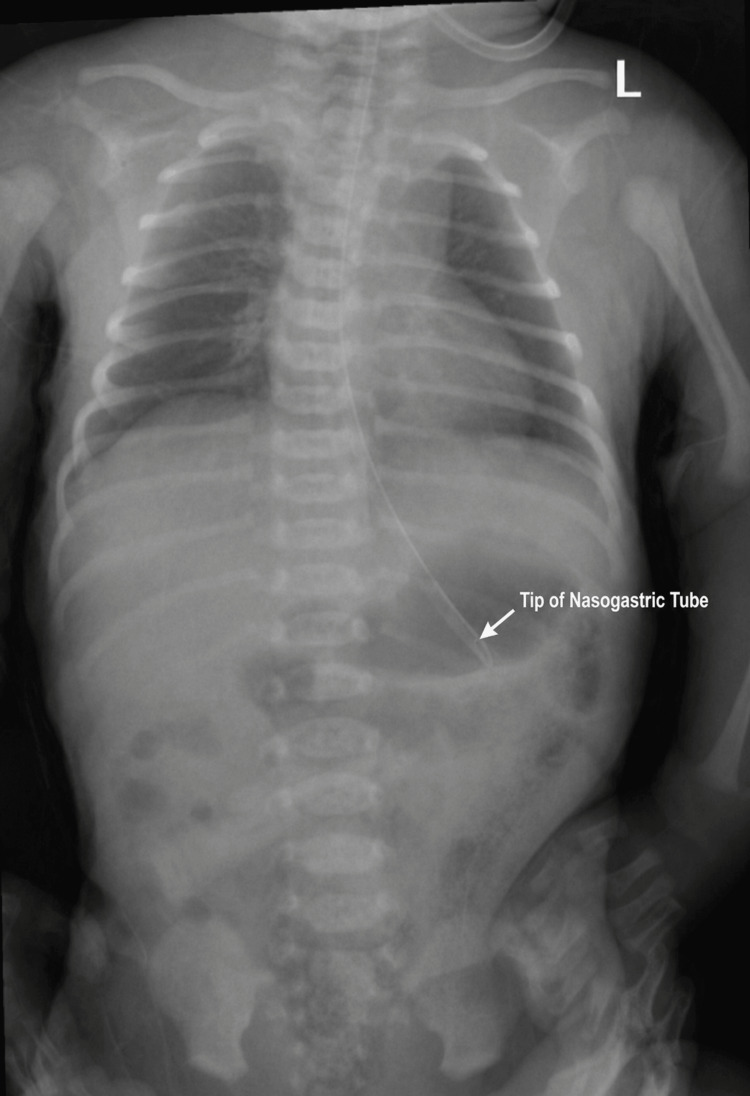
Initial anteroposterior chest and abdominal radiograph The radiograph shows very mild gaseous distension of the stomach and bowel loops. A nasogastric tube is seen, with its tip projecting over the stomach. No obvious pneumoperitoneum is identified.
